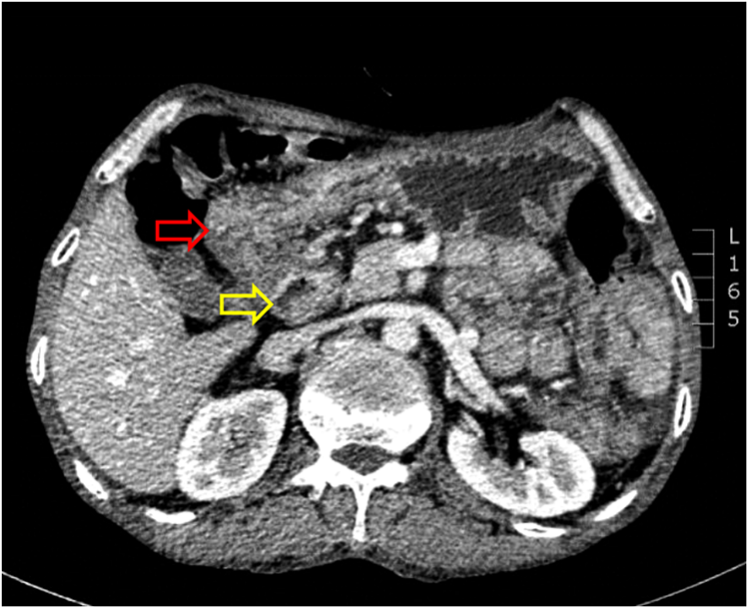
Computed tomography indicated no clear boundary between the descending duodenum and the transverse colon (the red arrow indicates the transverse colon, and the yellow arrow indicates the descending duodenum).
